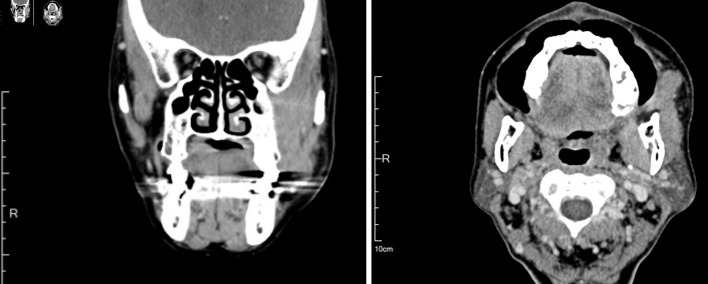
Contrast-enhanced computed tomography revealed an infiltrative soft-tissue mass measuring 3.1 × 1.5 × 3.5 cm, centered in the masticator space with effacement of fat planes between the masseter, pterygoid, and temporalis muscles.
